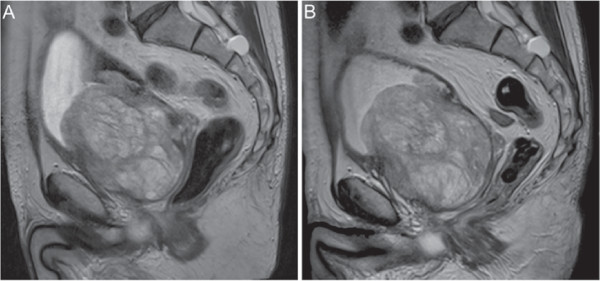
Sequential Saggital T2 MR Imaging of the Patient’s Giant Prostate. Image A - MRI from September 2008 (6 months prior to embolisation). Image B - MRI from April 2011 (25 months after embolisation). Note the minimal change in volume and signal density between the two images.
